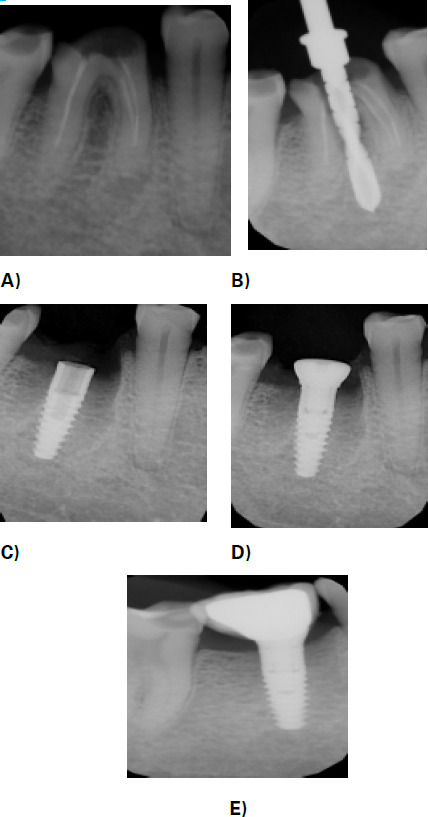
Radiographic image showing A) loss of coronal structure with linear radiopacity short of apex on both the roots along with file separation on mesial root, B) After osteotomy, checking for center point in between mesial and distal roots,C) Placement of implant on the drilled area after removing roots D) Gingival former placement on implant, E) Placement of crown on implant after 3 months.
